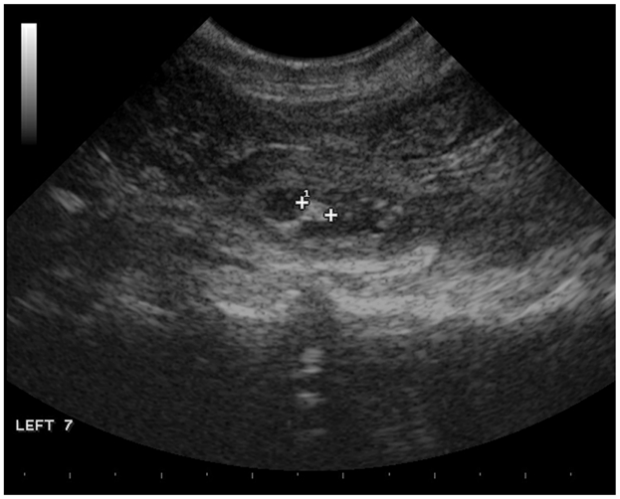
Ultrasound image showing an egg in the uterus of echidna 5D5E on July 23 2008. Fertilization probably occurred on July 9, but she had fresh sperm in her reproductive tract and was also torpid. Distance between the two markers showing the structure within the egg is 0.35 cm.
